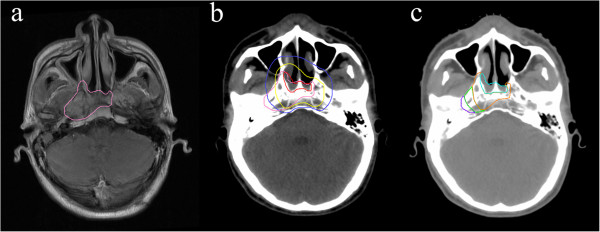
Delineation of target volumes. (a) The gross recurrent tumor volume (GTVr) was identified on MRI images. (b) The GTVr was transferred to the original planning CT. Red line is gross tumor volume (GTV); yellow line, CTV1; blue line, CTV2. (c) Cyan line, TG; orange line, TC1; green line, TC2; purple line, TE; TG+TC1+TC2+TE=GTVr.
